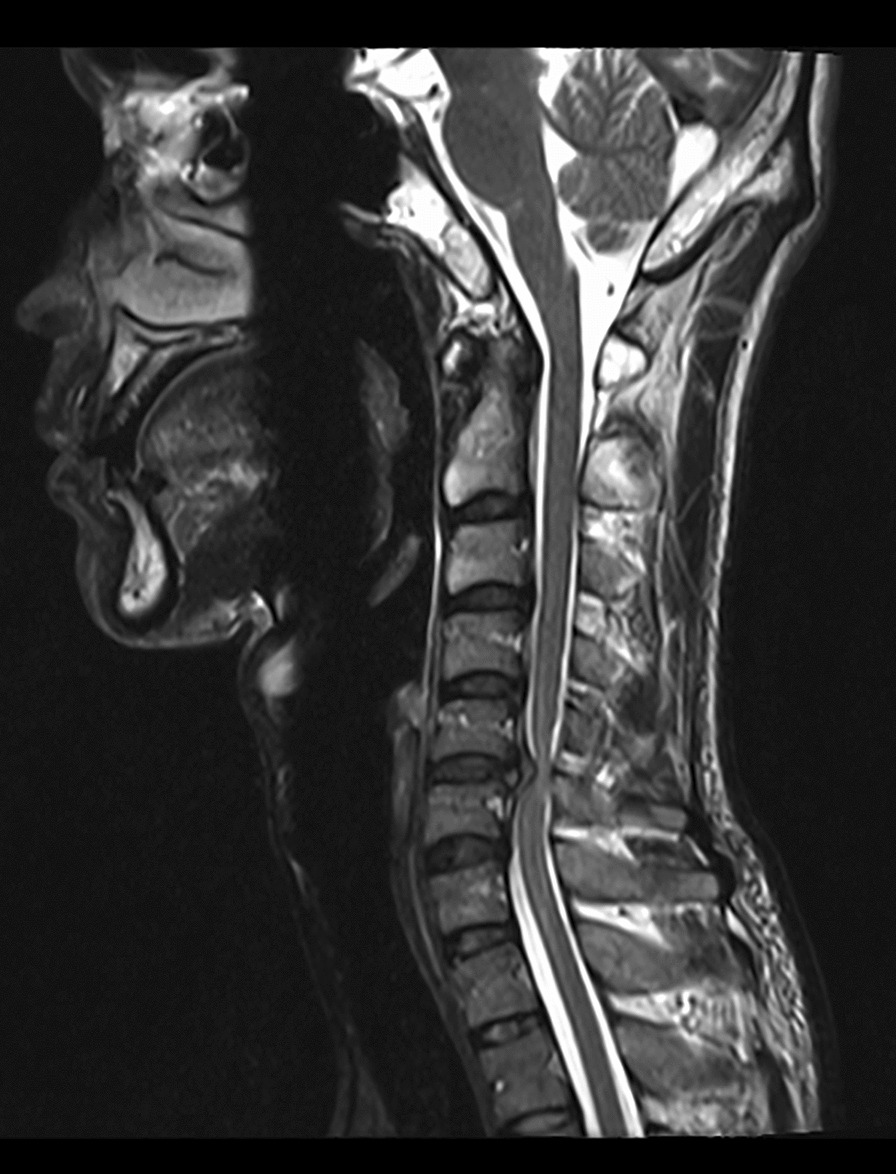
Preoperative MRI showed giant cervical disc herniation
